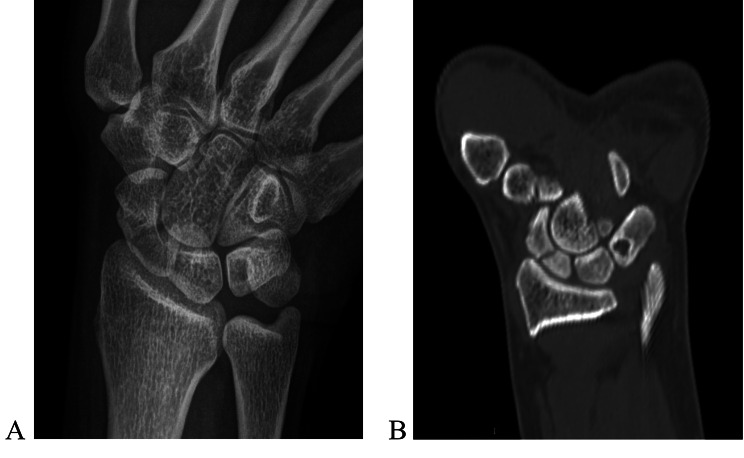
Preoperative radiograph (A) and coronal CT of the right wrist (B), showing a 35-year-old woman with a delayed-presentation scaphoid waist fracture 72 days after injury
